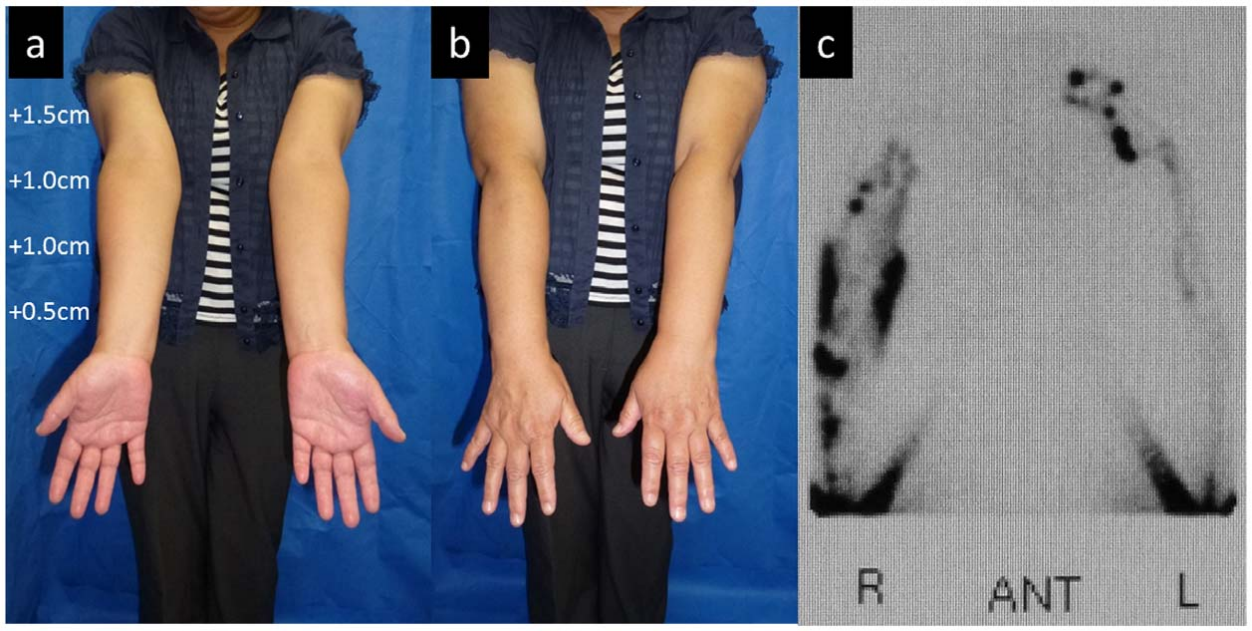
Case 3. (a, b) Edematous condition before LVA. Compared to the healthy side (left), the circumferences were increased by 1.5, 1.0, 1.0, and 0.5 cm for the upper arm, elbow, forearm, and wrist, respectively. (c) Findings from lymphoscintigraphy. Reflux in the skin (dermal backflow) was observed in the medial and lateral upper arm and lateral forearm in the right upper limb. A normal lymph vessel distribution was apparent in the left upper limb.
